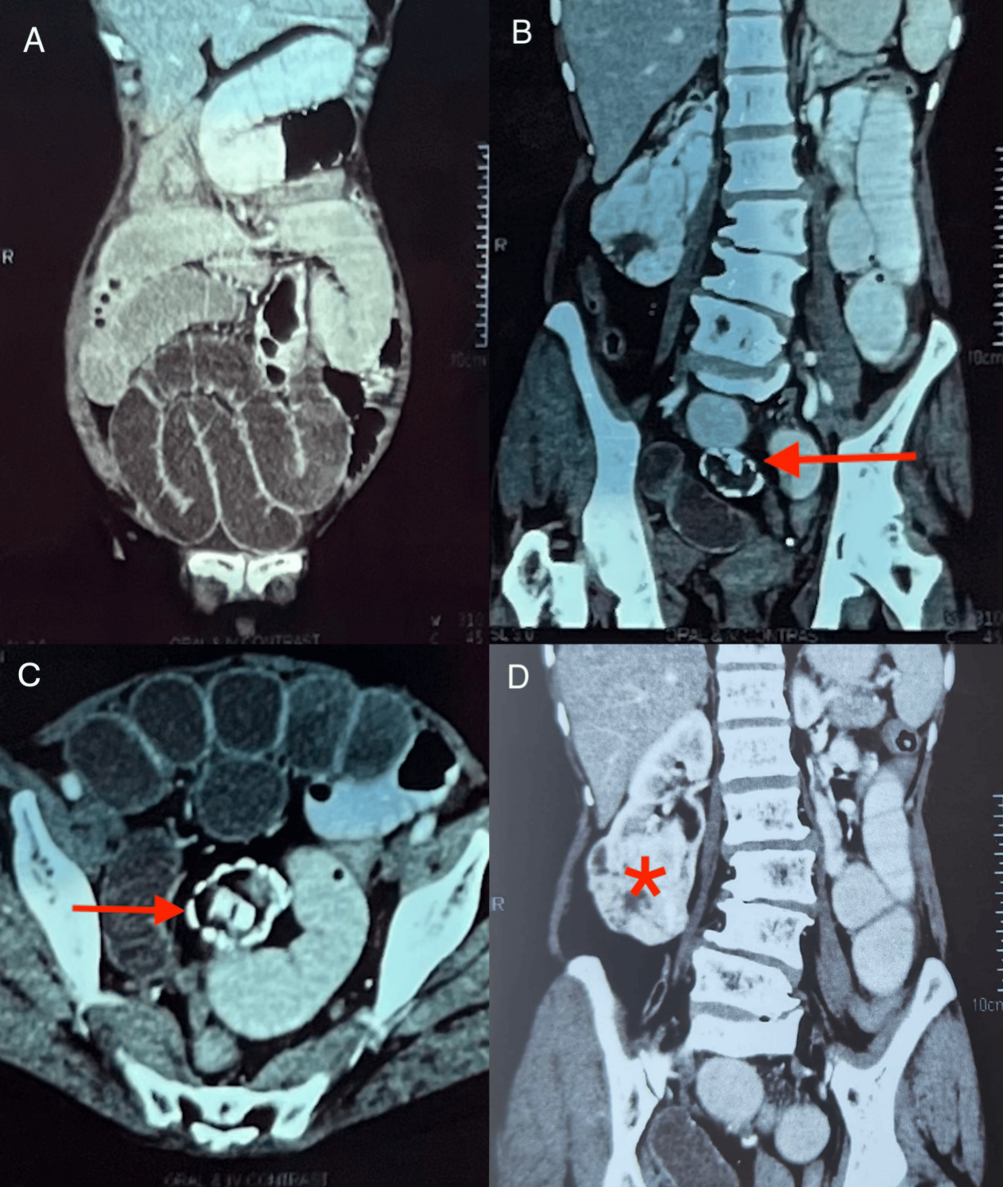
Abdominal CECT with oral and intravenous contrast (A) Coronal image showing dilated small bowel loops. (B and C) Coronal and axial views showing a heterogeneous mass (red arrow) with fat attenuation and peripheral calcification in the lower abdominal region abutting the dilated small bowel loops, suggestive of a superiorly displaced right adnexal mass. Mild sclerotic and degenerative changes in the lumbar vertebrae are present. (D) Coronal view showing a well-defined, heterogeneously enhancing exophytic solid mass lesion (asterisk) with necrosis in the lower pole of the right kidney CECT: contrast-enhanced computed tomography
